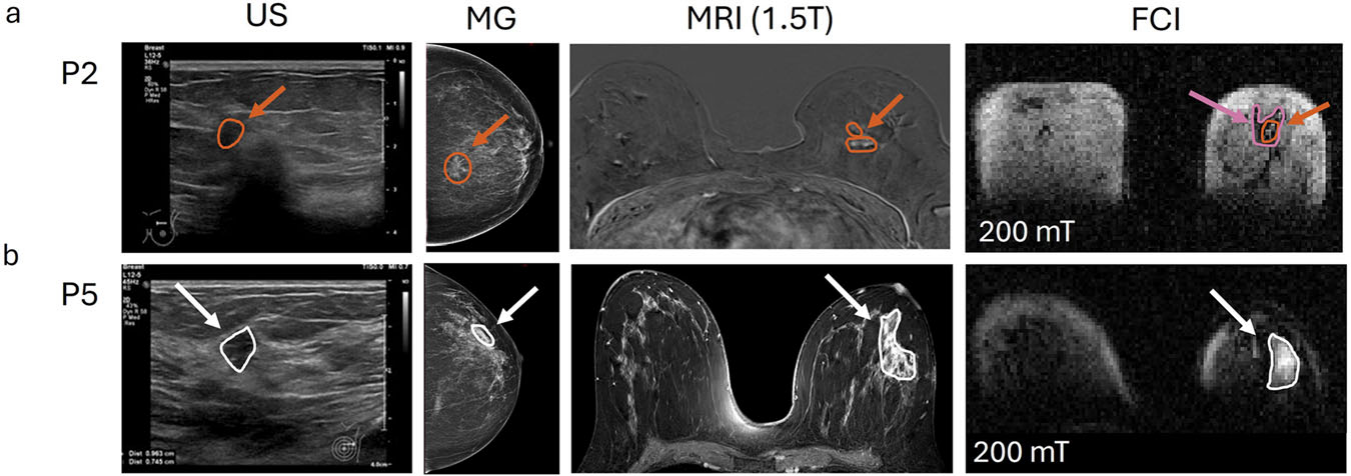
Comparison of images from US, MG, MRI and FCI. Ultrasound (US), Mammogram (MG), axial MRI and FCI images from volunteers P2 and P5. The ROIs highlight areas that correspond to the tumour. a P2 presented two distinct tumours in the left breast: ILC surrounded by DCIS. The ILC is detected by US and MG and is shown as bright areas in MRI (orange ROIs indicated by orange arrows). The DCIS is not seen in US, MG, and MRI. FCI detected both lesions with bright area corresponding to ILC and dark area corresponding to DCIS as indicated by orange and purple ROIs with arrows respectively in FCI images (breast was squeezed due to the restricted RF coil size). b For volunteer P5, the tumour is localised at the left breast, and it is indicated by white ROIs and arrows; For P5, the DCIS was not seen in MG but was retrospectively analysed to show a lesion of 25 mm. US showed a smaller mass of 11 mm. FCI detected the DCIS area (tumour in white ROI indicated by white arrows). (MG: Mammography, US: Ultrasounds, FCI: Field-Cycling Imaging, ILC: Invasive Lobular Carcinoma, DCIS: Ductal Carcinoma In Situ, ROI: Region Of Interest).
